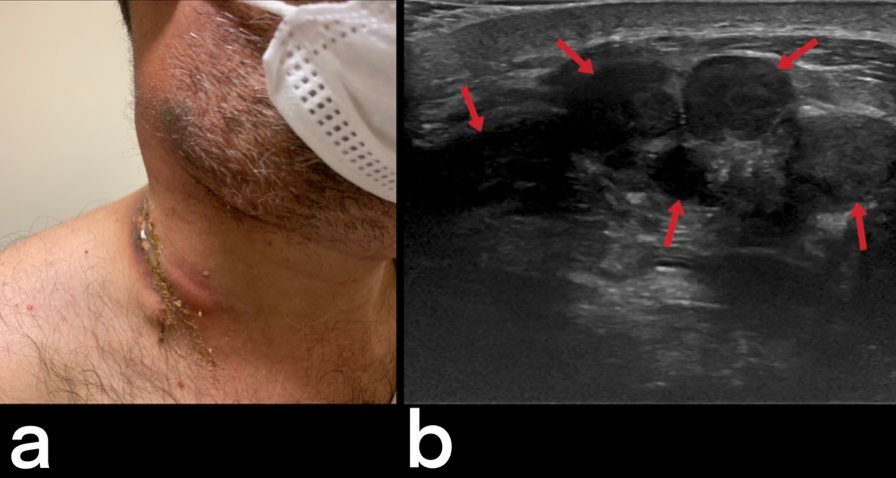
A 32-year-old male. Painless, discrete, freely mobile, fistulized lumps in the neck are grown progressively (a). Ultrasound showed multiple necrotic lymph nodes (b). Scrofula of the neck
